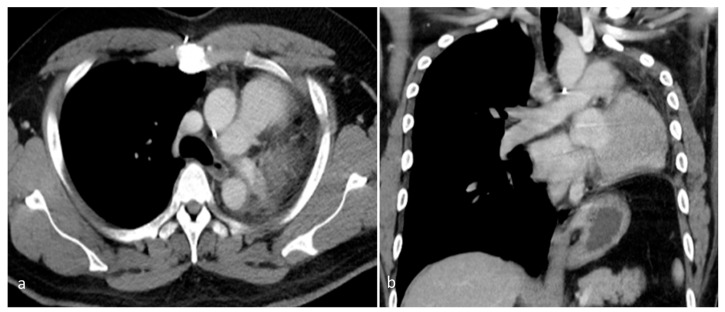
A contrast-enhanced chest CT, performed 2 years after surgery, revealed postoperative changes consistent with prior left pneumonectomy and the complete resection of the left pulmonary artery. (a) Axial view. (b) Coronal view. No residual or recurrent mass was apparent within the left hemithorax. The surgical bed appeared unremarkable, with no signs of local tumor recurrence or the abnormal enhancement of soft tissue. The right lung was well aerated, with no new parenchymal lesions or suspicious nodules. There was no evidence of contralateral lung involvement. No abnormally enlarged mediastinal or hilar lymph nodes were observed. These findings suggest a stable postoperative status with no radiological evidence of disease recurrence over 2 years after surgical resection.
